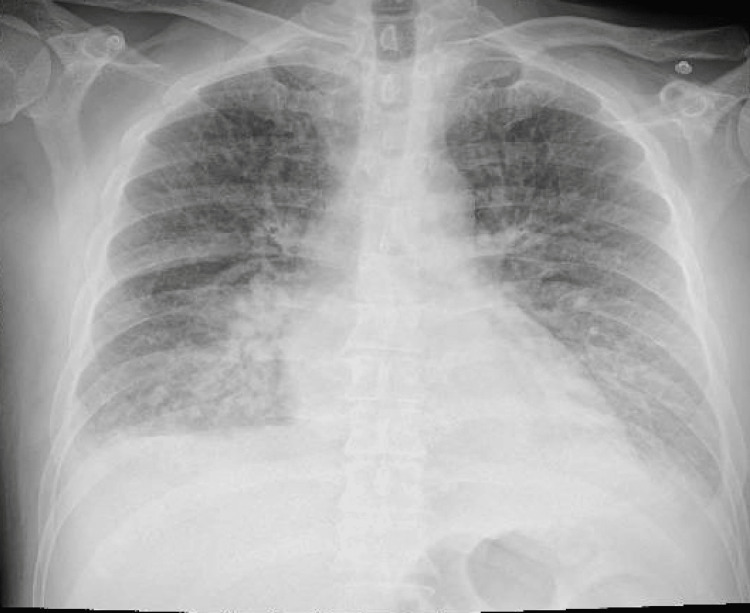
Pulmonary oedema on the anterior-posterior view of the chest X-ray.
